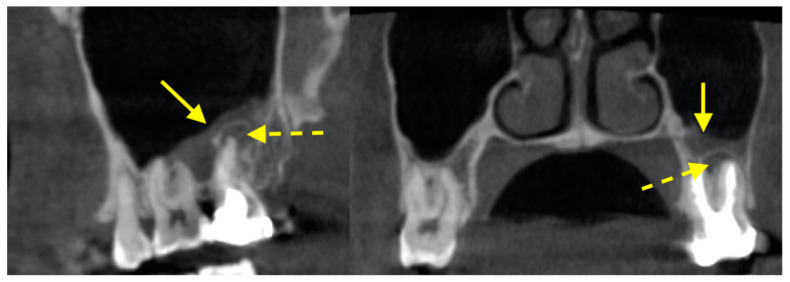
Sinus membrane thickening (solid arrow) in the left maxillary sinus associated with a periapical lesion (dotted arrow).
